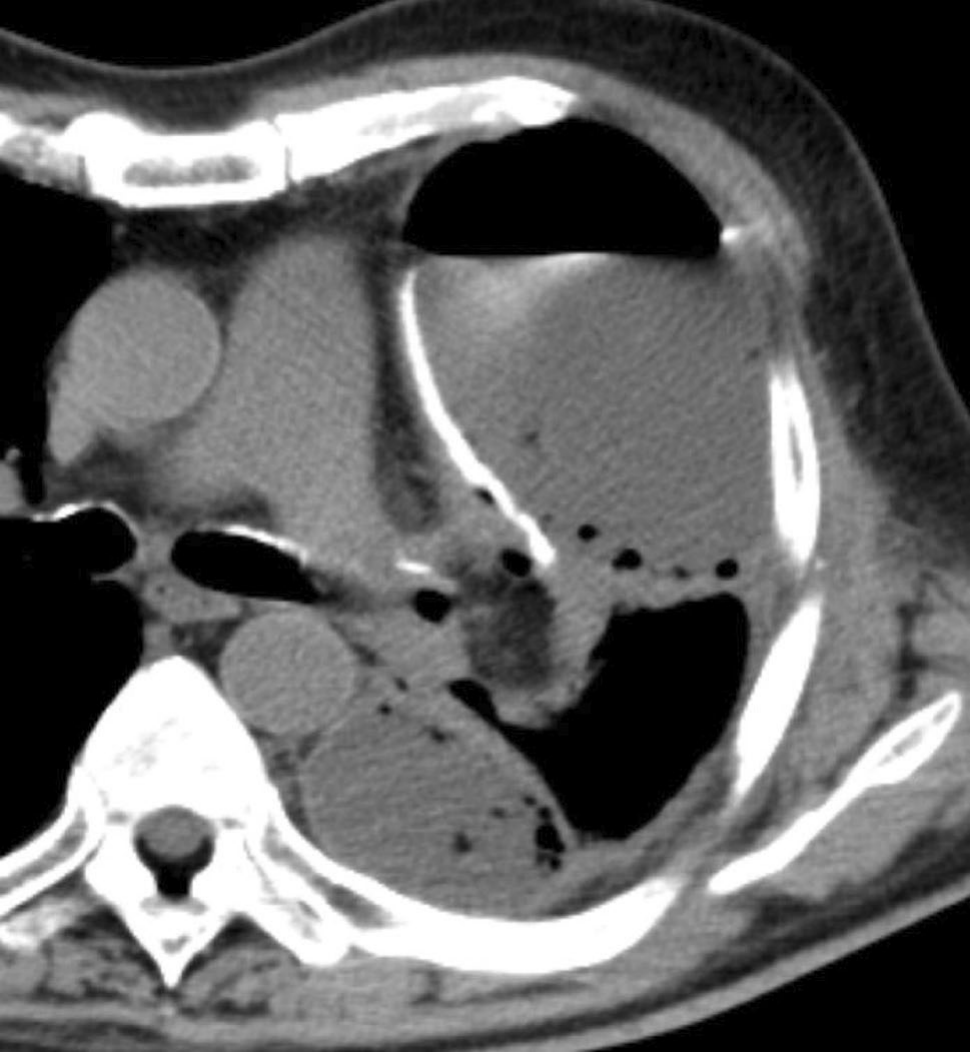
Empyema following left upper and lower segmentectomy to bronchopleural fistula. CT reveals fluid accumulation in the left pleural cavity, accompanied by adhesions and intrapleural air, resulting in a convex pleural contour
